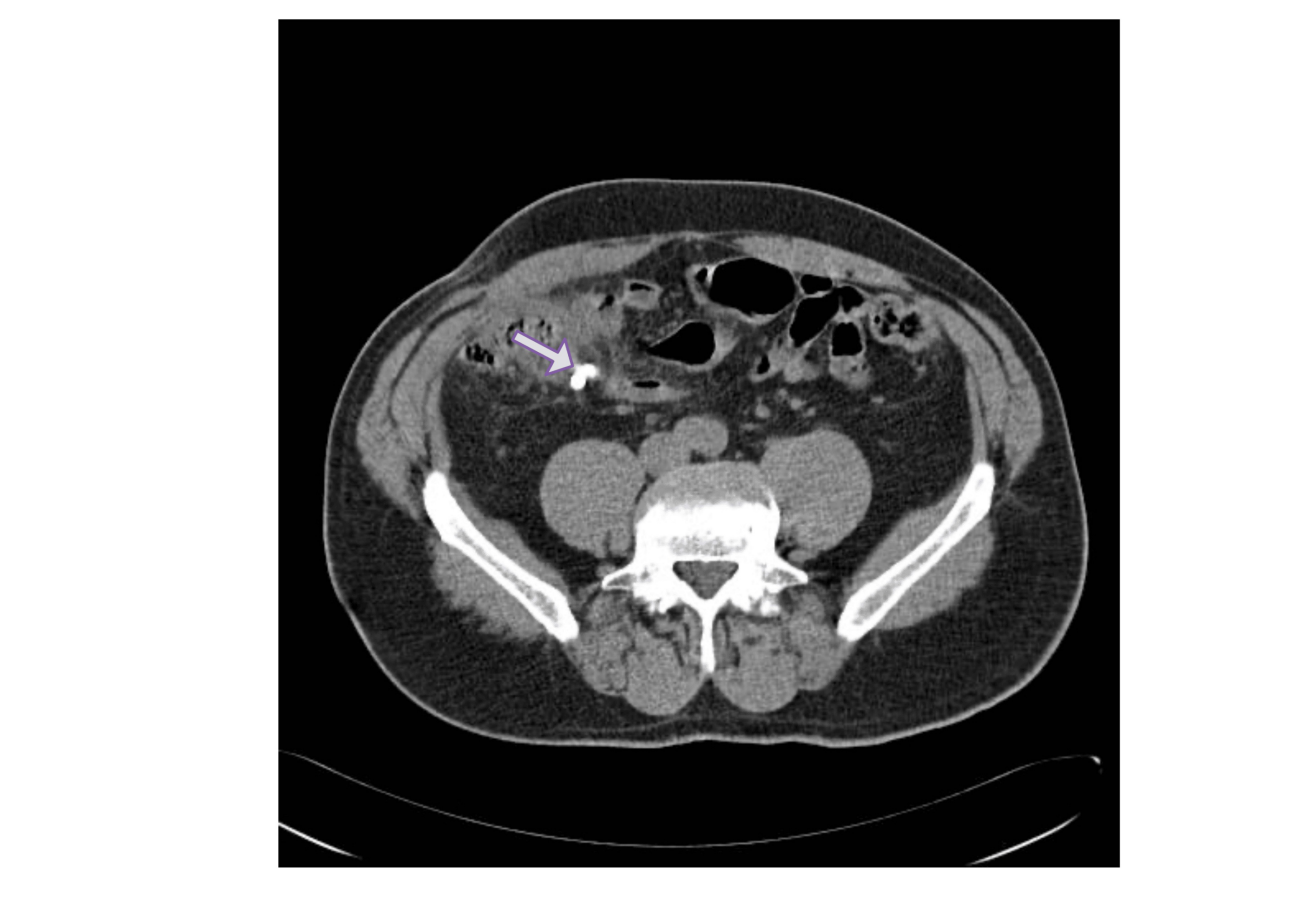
Computed tomography image demonstrating an inflamed appendix with an appendicolith (arrow) in the right lower quadrant Image adapted from Radiopaedia.org under Creative Commons Attribution-NonCommercial-ShareAlike license (CC BY-NC-SA 3.0) [11].
